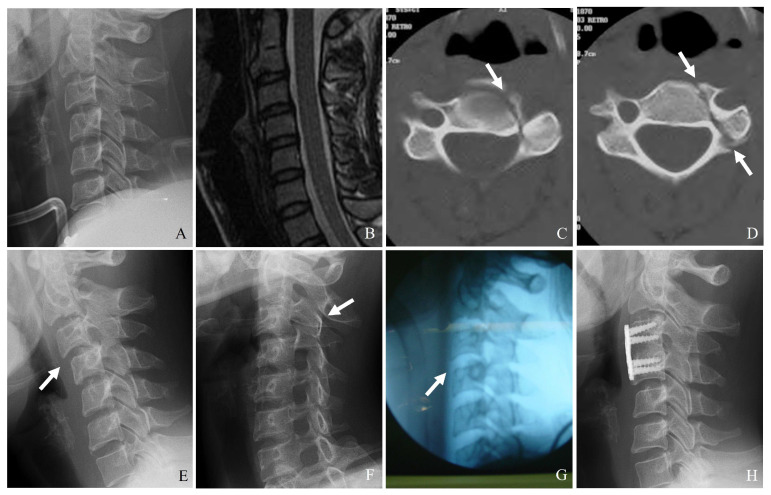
Plain X-ray (A) and magnetic resonance imaging (B) show no definite abnormalities. However, computed tomography (C,D) reveals a left facet fracture and fractures of the pedicle and body of C4 (white arrows). Delayed unilateral facet subluxation of C3–C4 occurred 1 month after wearing a rigid brace (white arrows) (E,F). The patient underwent closed reduction (white arrow) anterior cervical discectomy and fusion of C3–C4 (G,H).
